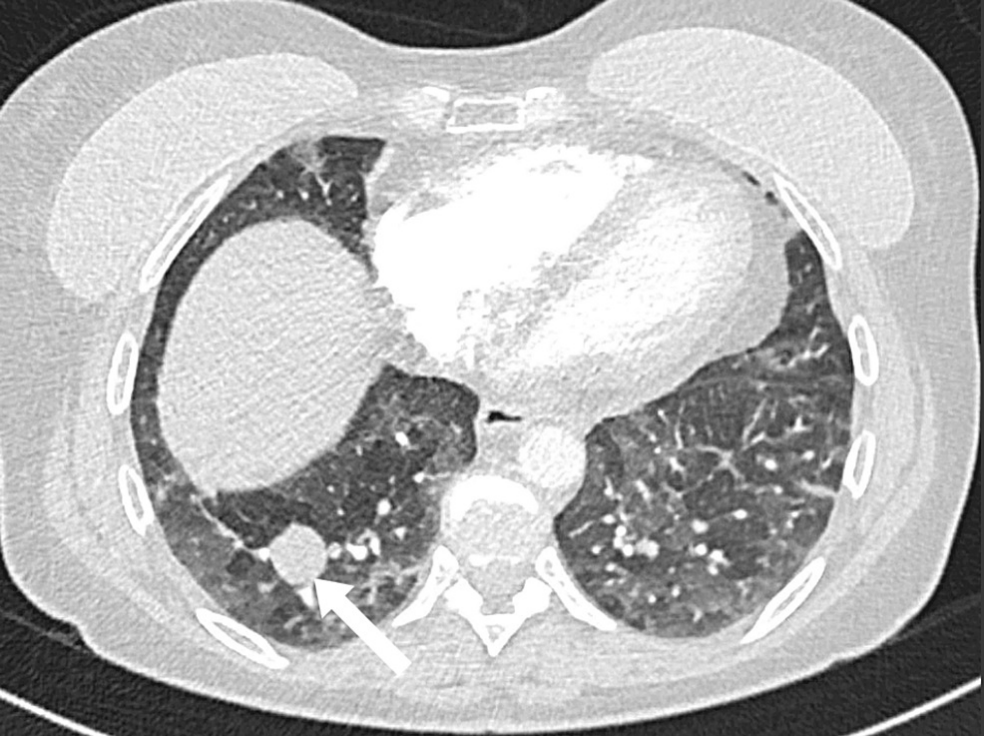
CT scan of right lower lobe nodule (1.9 x 1.9 cm)
